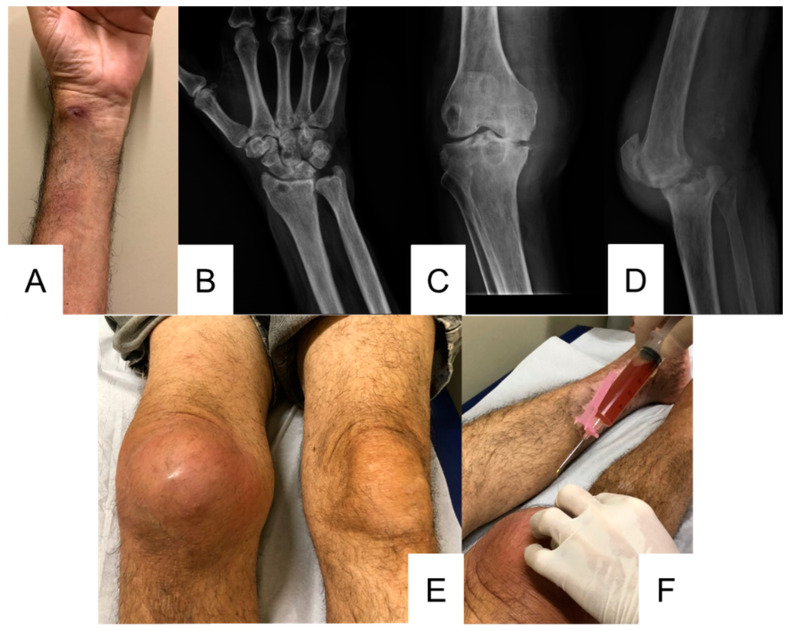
Wrist and knee osteoarticular sporotrichosis. (A) Nodule-ulcerative lesion on the left wrist (August 2018). (B) Radiograph of left wrist showing osteolytic lesions on the distal radius and capitate (July 2020). (C,D) Radiographs of the right knee showing tibial plateau erosions, joint space reduction, and irregular osteolytic lesions present on both the femur and tibia (August 2018 and August 2020, respectively). (E) Right knee with swelling, erythema, pain, and heat (August 2018). (F) Right knee synovial fluid aspiration puncture (August 2018).
